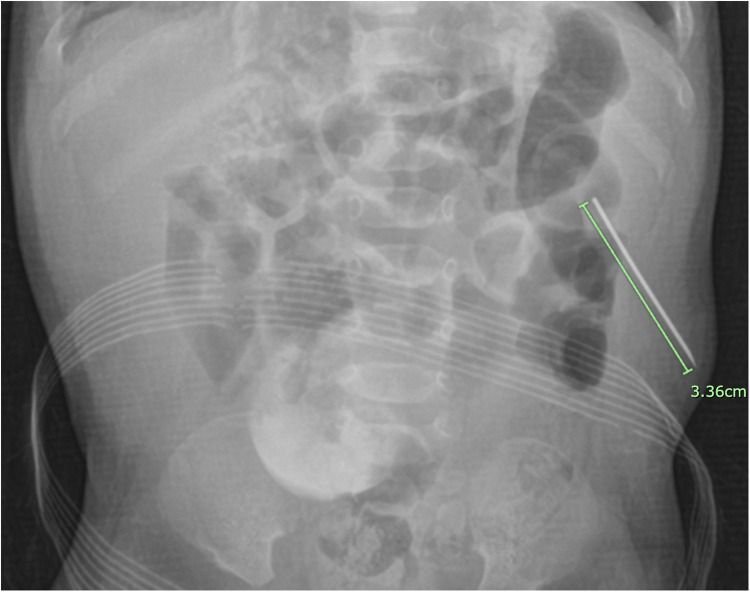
Plain abdominal radiograph demonstrating a thin, elongated needle-like foreign body oriented obliquely in the left flank, measuring 3.36 cm in length (green line).
